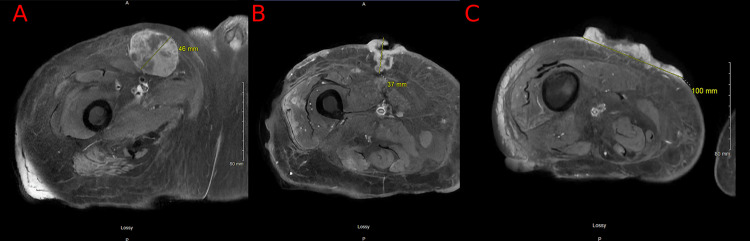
Magnetic resonance imaging of the right femur showing enlarged right inguinal lymph nodes (A) and deep skin and subcutaneous soft tissue lesions in the right thigh (B, C)
